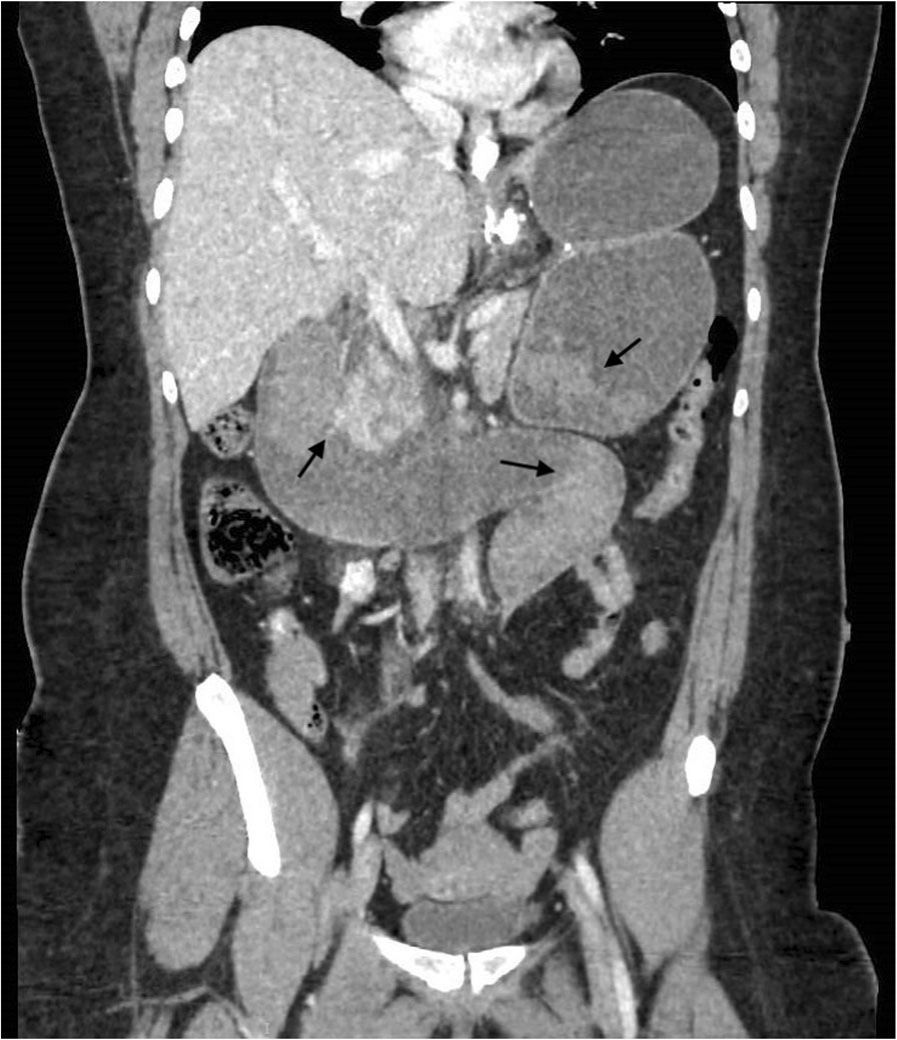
CT abdomen on the day of presentation. The CT-scan shows dilatation of the excluded stomach and of the proximal small intestines with hyperdensity (arrow), which appeared to be intraluminal blood clots during laparoscopy
